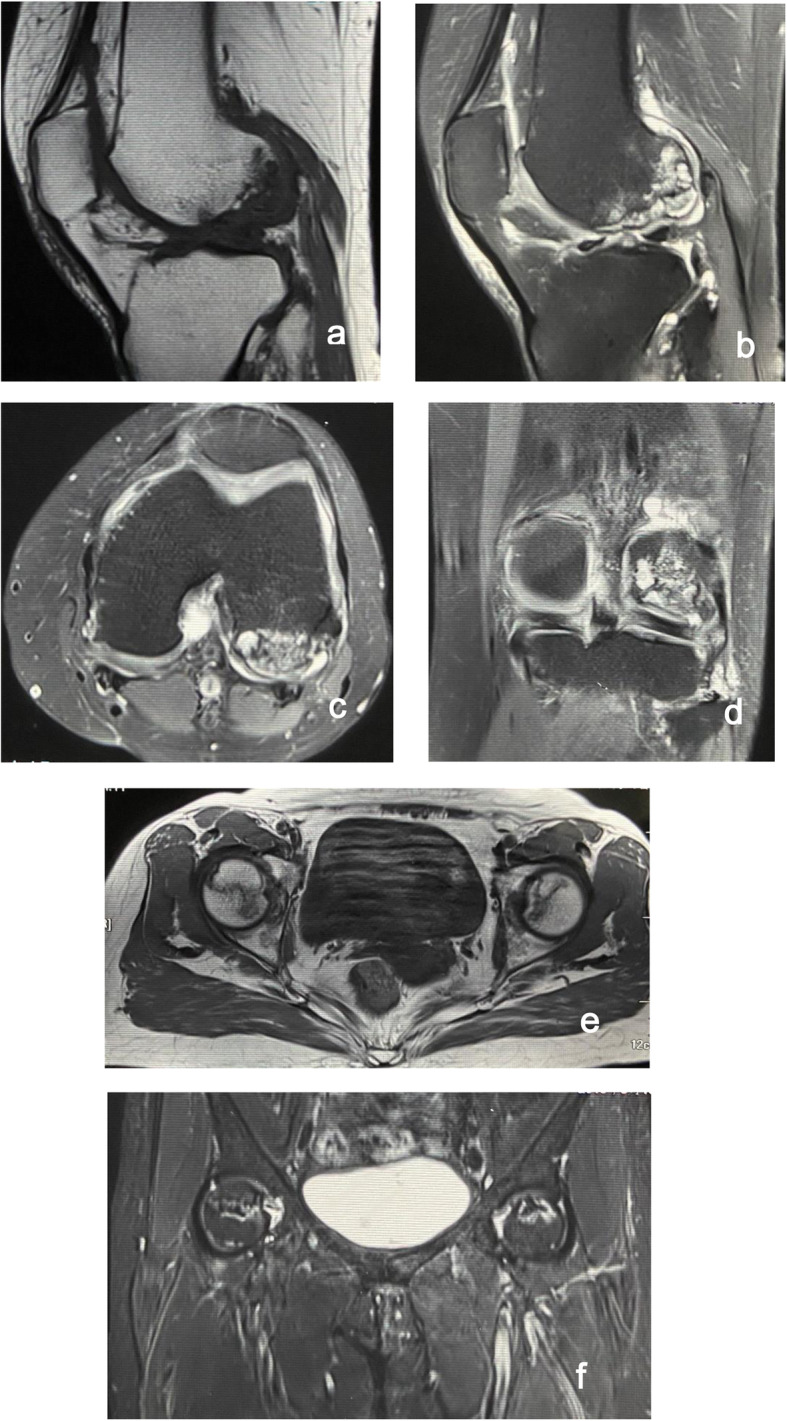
(a) Sagittal T_1_-weighted MRI showing a low-signal subchondral lesion of serpiginous morphology. (b, c, d) T_2_-weighted sequences demonstrated a serpiginous lesion of a relatively disorganized area of edema with hyperintense signal, and focal epiphyseal contour depressions. (e) Axial T_1_-weighted MRI showing a bilateral large subchondral lesion of isointense. (f) Coronal T-weighted MRI showing necrotic fragments in bilateral femoral head, with mixed increased and reduced signal intensity
